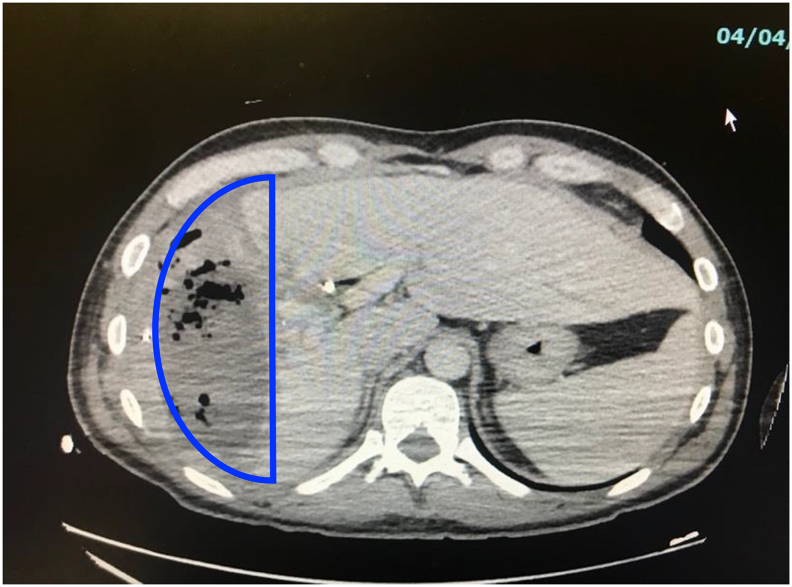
CT scan showing giant hepatic abscess with occult bile leak. The outlined arch indicates the liver nestled within the ribs, preventing natural wound contractile forces.
